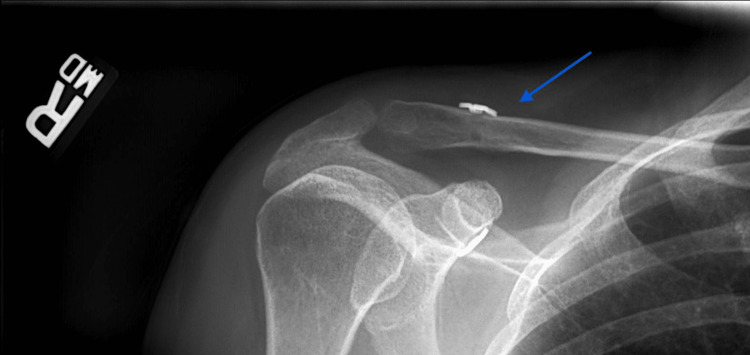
Immediate postoperative radiograph of a right shoulder demonstrates acromioclavicular reconstruction (blue arrow) with a single coracoclavicular tunnel
